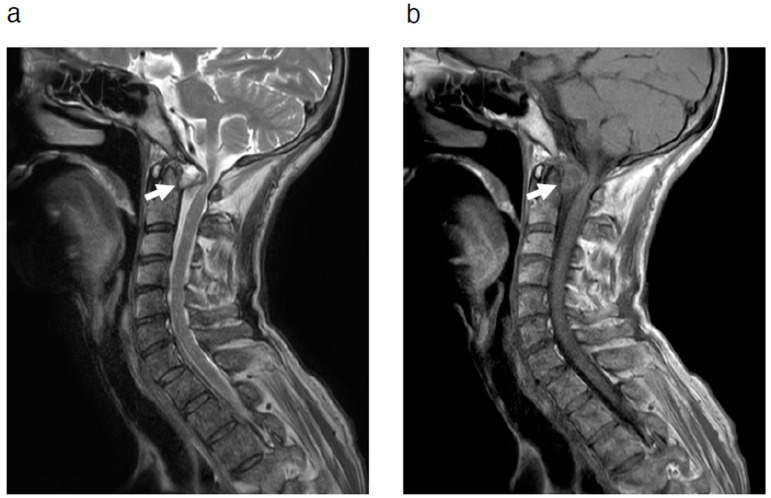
MRI at the initial visit revealed a retro-odontoid pseudotumor (white arrows). Sagittal view of T2-weighted (a) and T1-weighted (b) images. MRI = magnetic resonance imaging.
